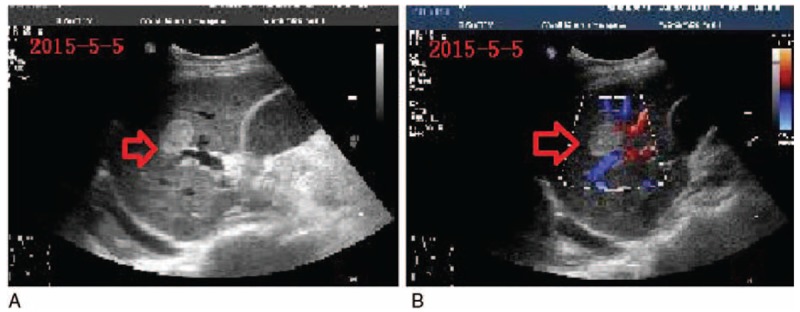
Ultrasonography showing multiple masses in the liver (red arrow). (A) A two-dimensional ultrasound. (B) Color Doppler ultrasound.
